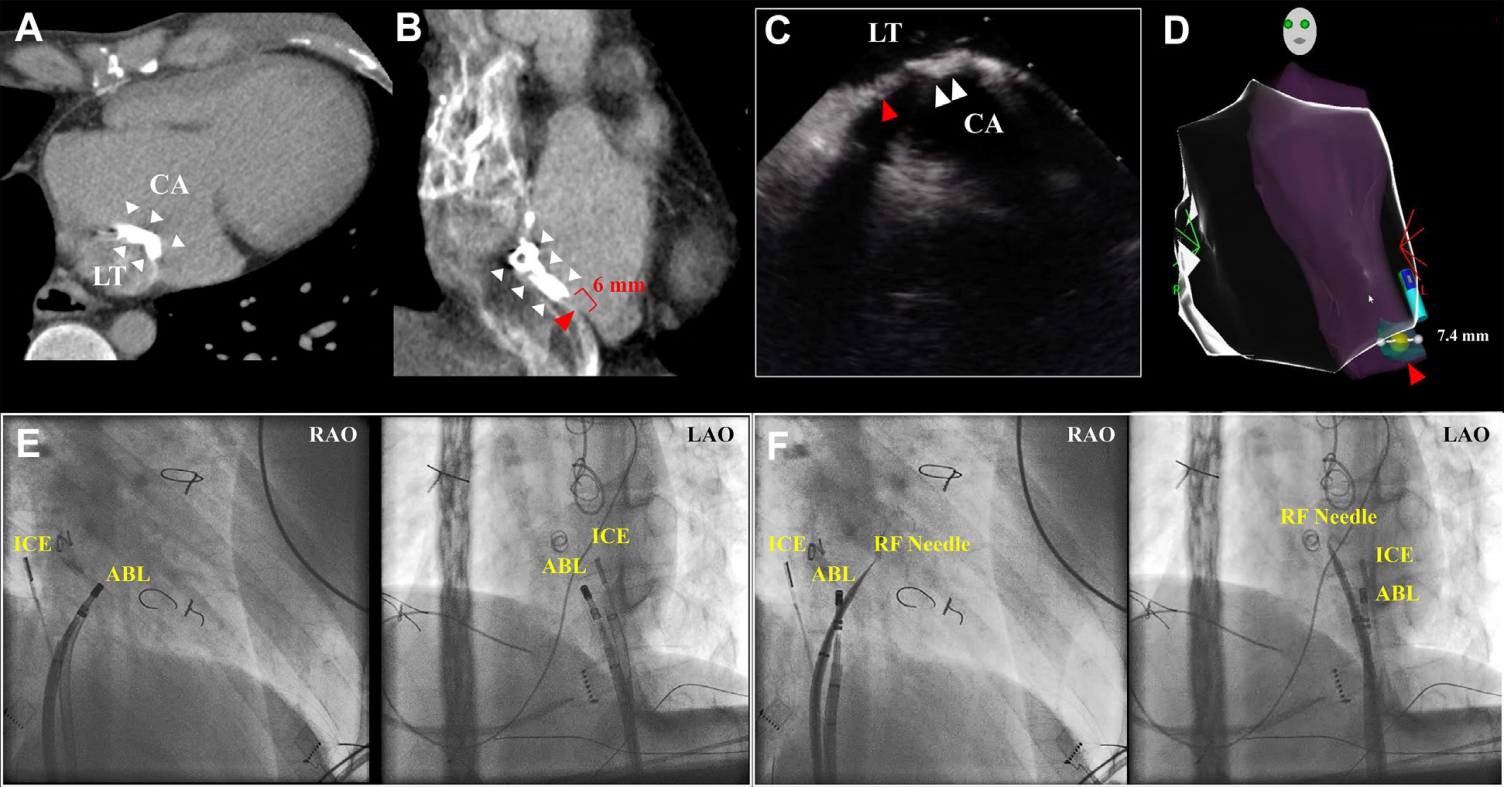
(A, B) Computed tomography before the ablation. A highly calcified lateral tunnel (LT) baffle (white arrowhead) and adjacent native tissue whose length was 6 mm (red arrowhead) were observed. (C, D) Intracardiac echocardiography and the geometry constructed with SOUNDSTAR. A small normal myocardium channel (C, red arrowhead) adjacent to the highly calcified baffle was observed. A 3D map of the LT (D, pink area) and normal myocardial area (D, light green area) was created using SOUNDSTAR. The width of the normal myocardial area was 7.4 mm. That point is marked on the 3D map. (E) Using an ablation catheter, the puncture site was marked on the fluoroscopic image based on the 3D mapping. (F) Based on the marked site of the ablation catheter, a transbaffle puncture was performed using a radiofrequency needle. ABL, ablation catheter; CA, calcification; ICE, intracardiac echocardiography; LAO, left anterior oblique: RAO, right anterior oblique; RF, radiofrequency transbaffle.
